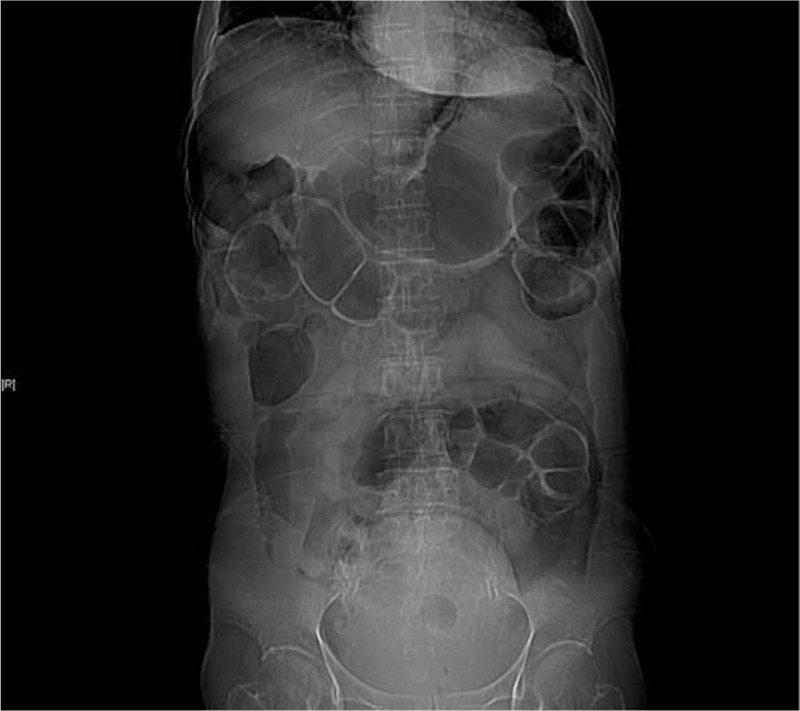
Abdominal CT shows gaseous distention of the intestine.
